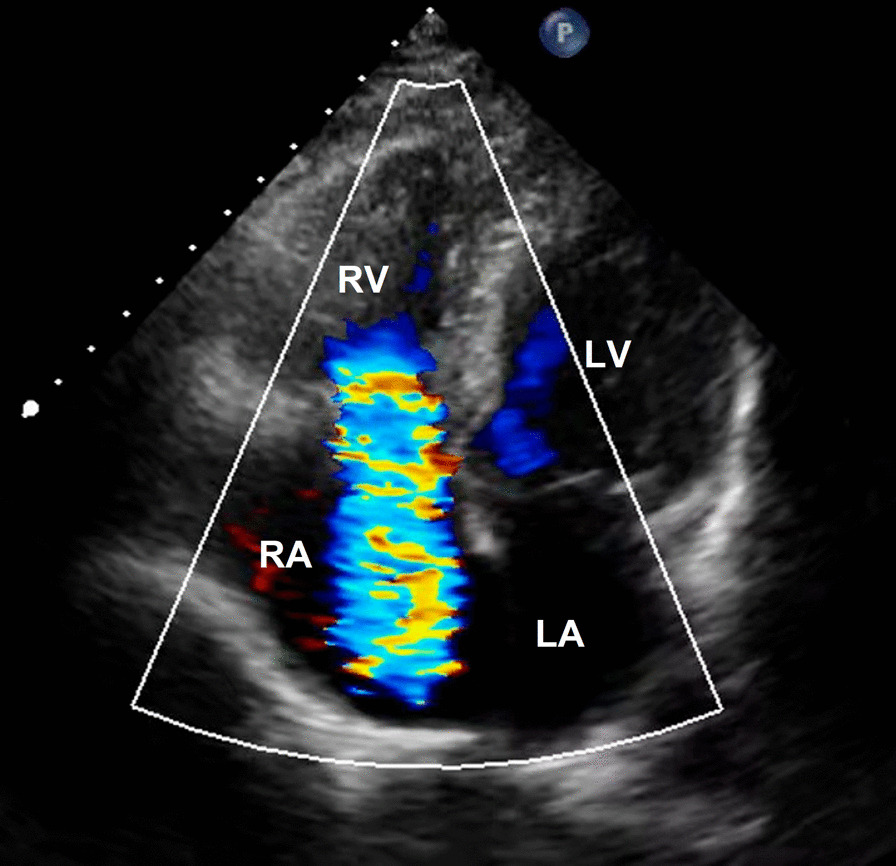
Transthoracic echocardiography shows severe tricuspid regurgitation. LA: Left atrium, LV: left ventricle, RA: right atrium, RV: right ventricle
